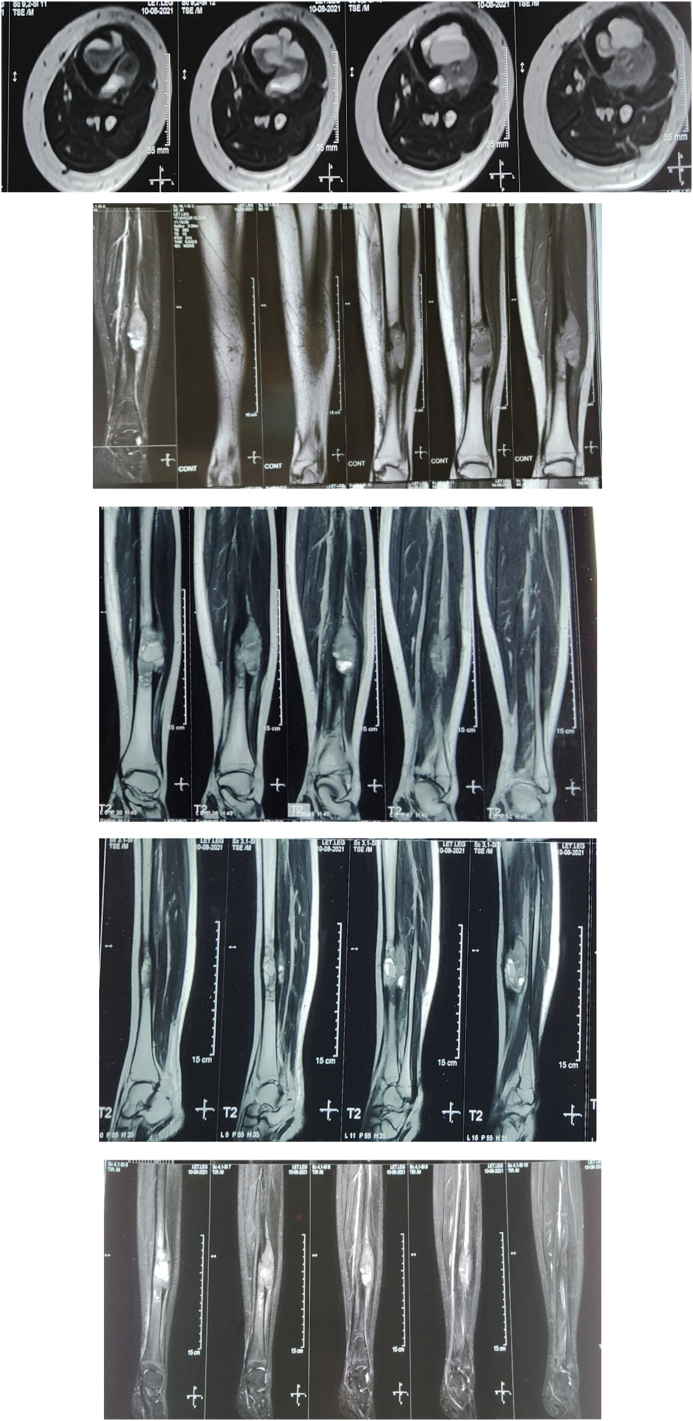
MRI of left tibia showing revealed mixed type of altered signal intensity, expansible lobulated cortical lesion in lower diaphysis of left tibia, showing heterogeneously hypo signal intensity on T1WI and heterogeneously hyper signal intensity on T2WI and STIR images with internal fluid level. The lesion displaced the adjacent muscle. After IV contrast, heterogeneous contrast was seen in the lesion. Subcutaneous soft tissue shows normal signal intensity without focal lesion.
